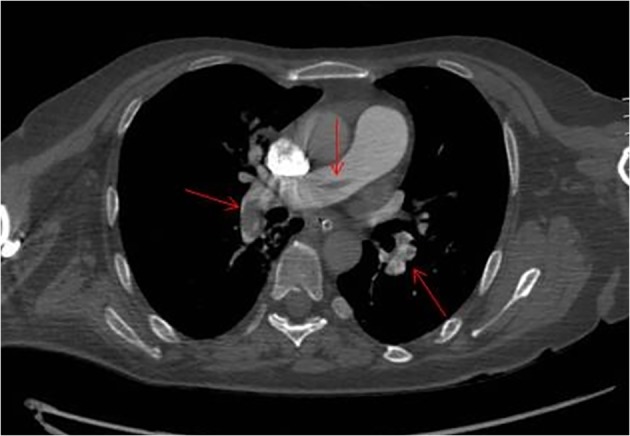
Pulmonary CT angiography axial showing multiple filling defects due to pulmonary artery embolism.
